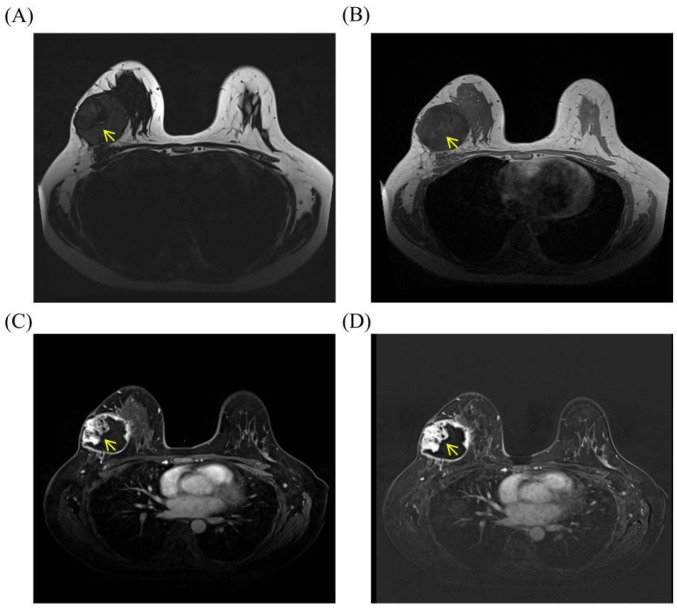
MRI findings of Case 1. (A) Axial T2-weighted fat-suppressed image (T2 Dixon water-only image) demonstrates a predominantly cystic mass in the upper outer and lower outer quadrants of the right breast, with an irregular inner wall, multiple mural nodules, and heterogeneous internal high- and low-signal components. (B) Axial pre-contrast dynamic T1-weighted image shows mural nodules along the cyst wall before contrast administration. (C) Axial early post-contrast dynamic image demonstrates conspicuous enhancement of the mural nodules and irregular cyst wall. (D) Axial subtraction image further confirms true enhancement of the mural nodules and cyst wall, supporting the presence of intracystic solid components rather than posterior background parenchymal enhancement.
